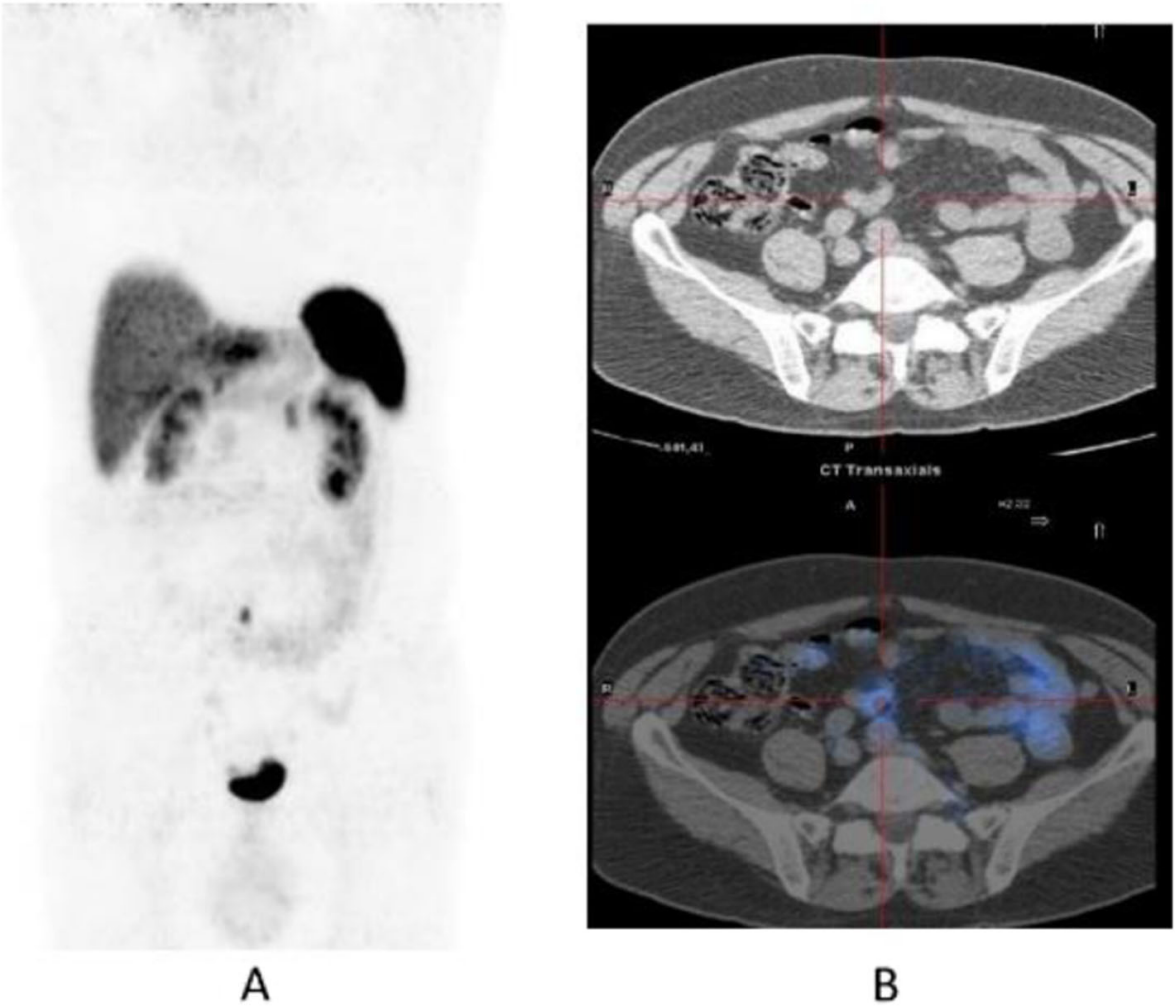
68Ga-DOTA peptide, suspected lesion of the liver, specificity. *Clinical history*: 52 y.o. male with an incidental detection of a single liver lesion in the left lobe. The lesion is suspect to be a metastasis on ultrasound and CT from an unknown primary site. *PET/CT findings*: increased focal uptake in a loop of the ileum, suspect to be a primary tumour. Increased uptake in the left liver lobe consistent with a secondary lesion (a MIP, b CT and fused images). After surgery, the lesions were diagnosed to be a well-differentiated NET grade 1 (Ki67 2%)
